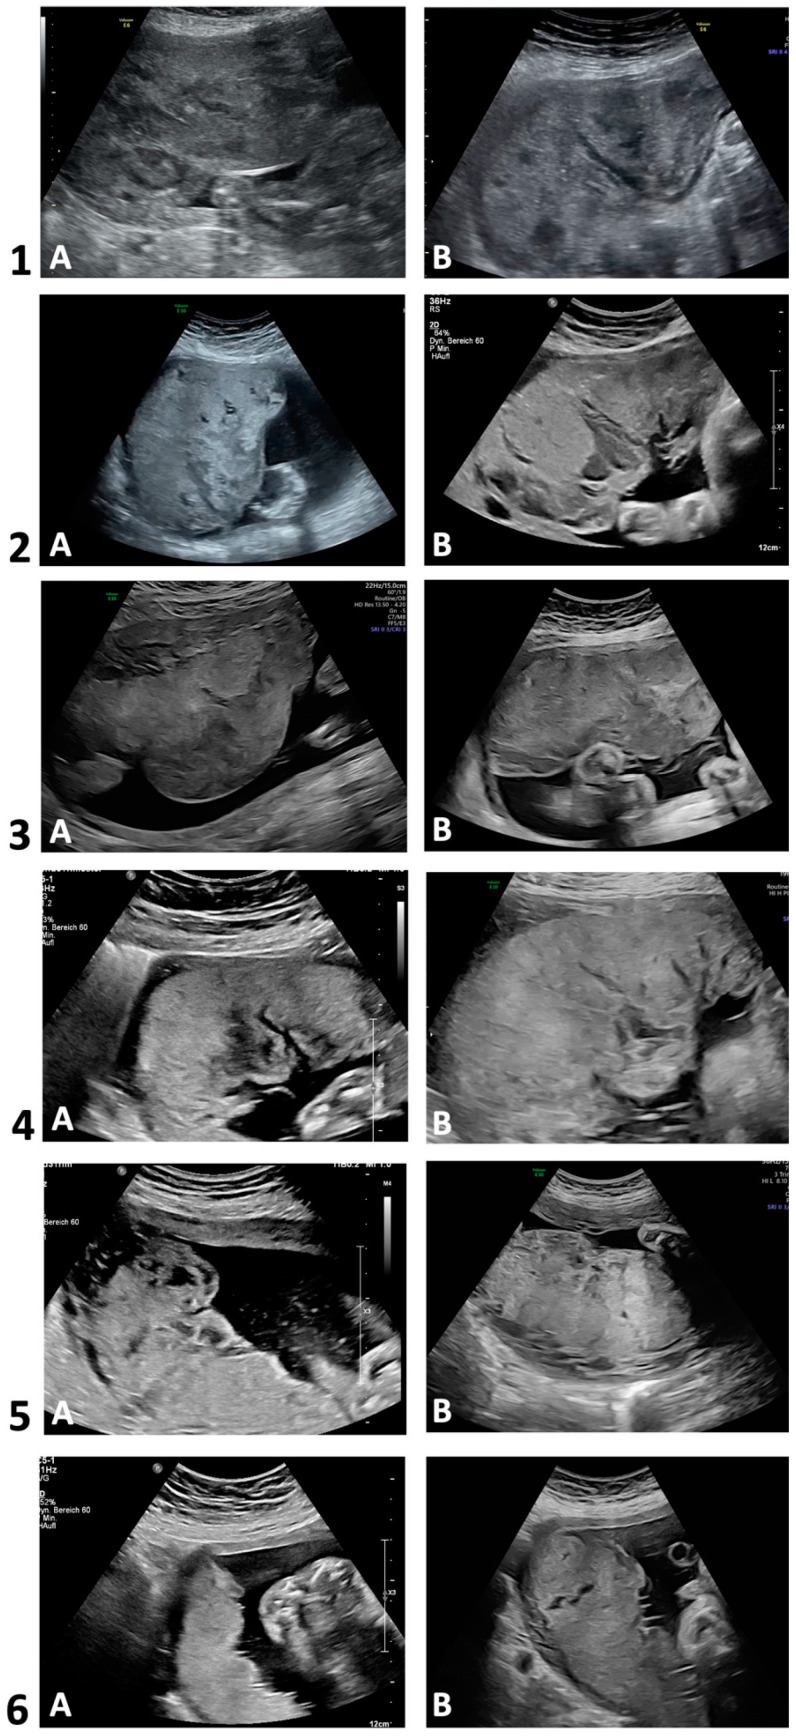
Transabdominal ultrasounds in 2D grayscale. Development of placental sonomorphologic appearance of cases with Fontan circulation (n = 6). Case 1 (A: 25 + 5; B: 32 + 1), case 2 (A: 29 + 4; B: 36 + 3); case 3 (A: 20 + 5; B: 30 + 5); case 4 (A: 23 + 6; B: 25 + 0); case 5 (A: 22 + 1; B: 32 + 1); case 6 (A: 24 + 0; B: 34 + 6). Gestational age (week + day).
